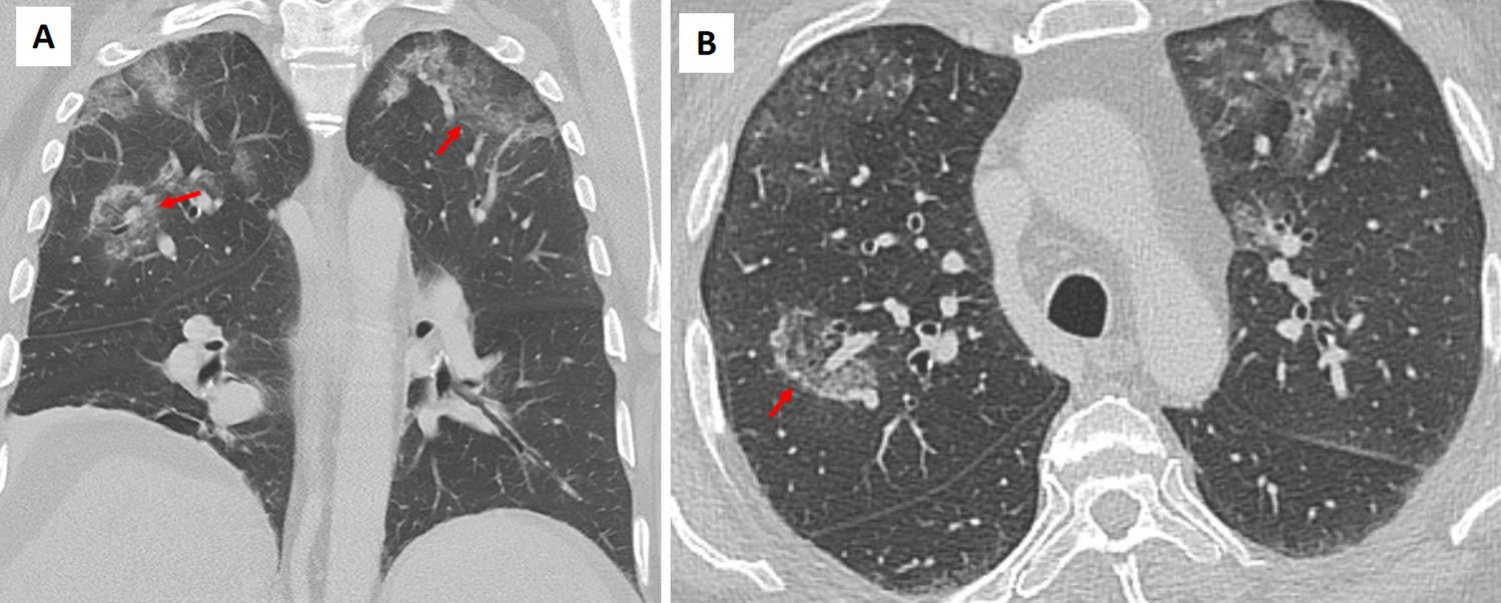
A Coronal and (B) axial chest CT images demonstrate upper lung-predominant ground-glass opacity (GGO) and the reverse halo sign (arrows) in a patient diagnosed with eosinophilic pneumonia. The patient had peripheral eosinophilia of 1264 eosinophils/μL (normal range ≥ 500 eosinophils/μL)
